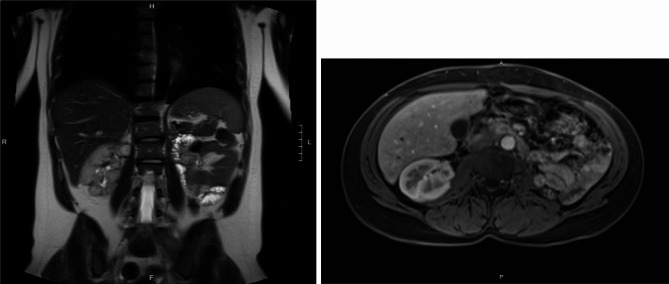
MRI abdomen with and without intravenous contrast performed after 1 year of antibiotic therapy. Imaging is notable for absent left kidney and improvement in right kidney morphology
